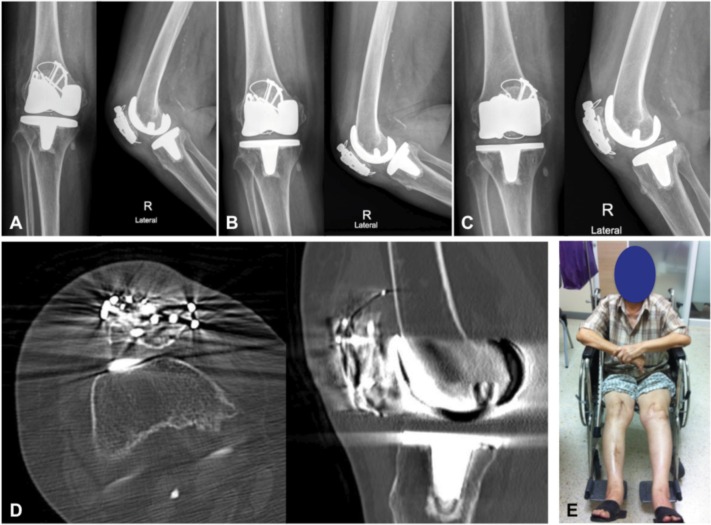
Postoperative radiographs and outcome; Postoperative follow-up radiographs on the first day (A), six months (B), and eighteen months (C) showed stable fixation without fracture displacement. On 6-month follow-up period, computerized tomography (D) demonstrated fracture healing as cortical continuation and disappearance of fracture line and the patient could fully extend her right knee similar to the normal side (E).
